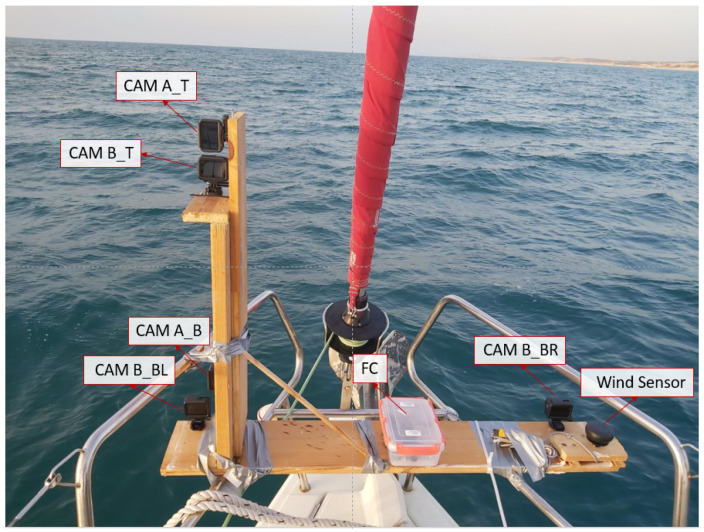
Multiple-camera wide-baseline stereo system located on an Elan 40 yacht during a field experiment. The stereo system is based on Go Pro cameras (Hero Black 7) and consists of 2 camera arrays: (*CAM-A*) two cameras, vertically displaced from each other. (*CAM-B*) 3 L-shaped cameras. Additional sensors: FC and ultrasonic wind sensor for future applications.
